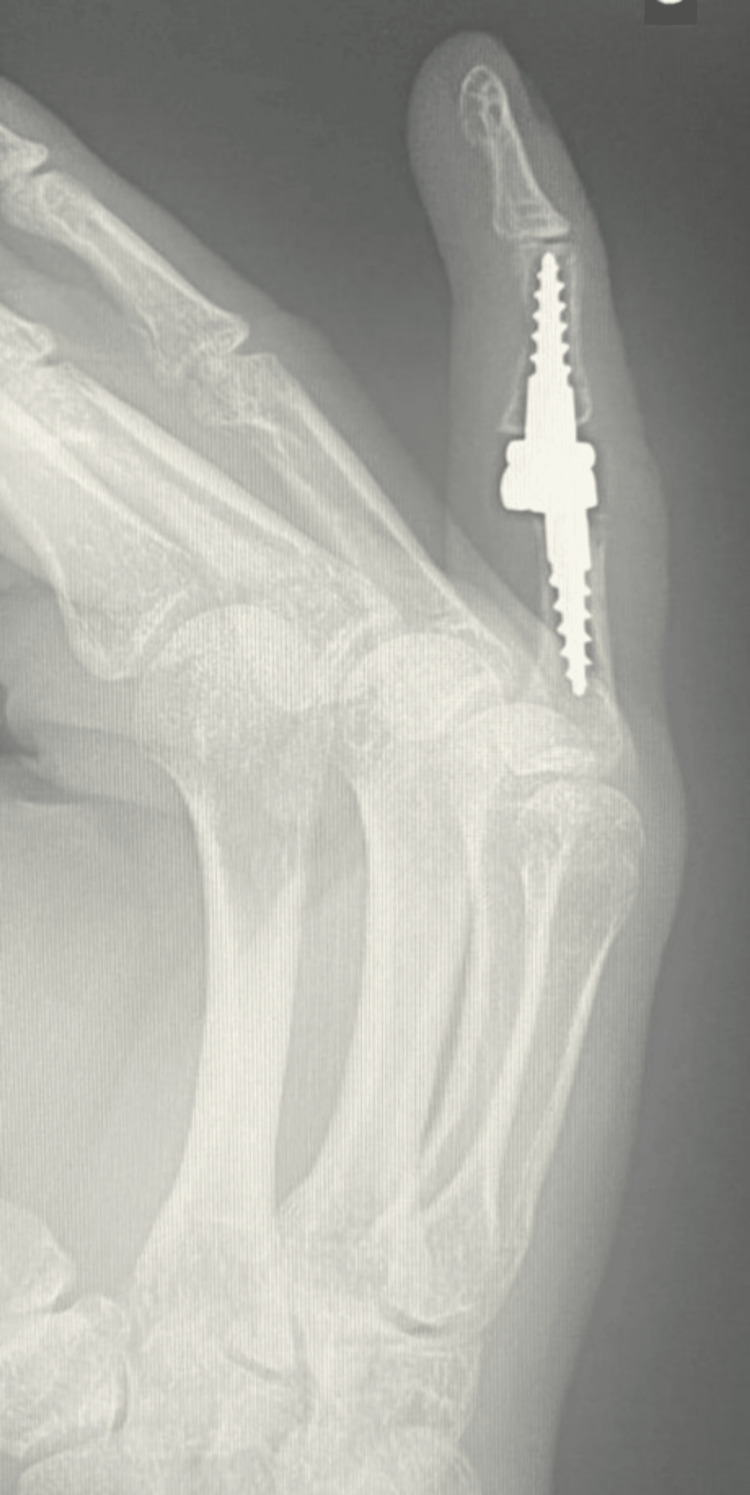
Right hand X-ray in lateral projection at week 21 of follow-up
